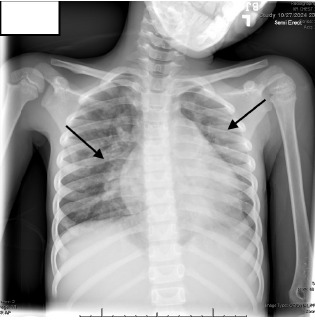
Chest radiograph showing bilateral infiltrate (arrow) in six-year-old child diagnosed with infective endocarditis.
